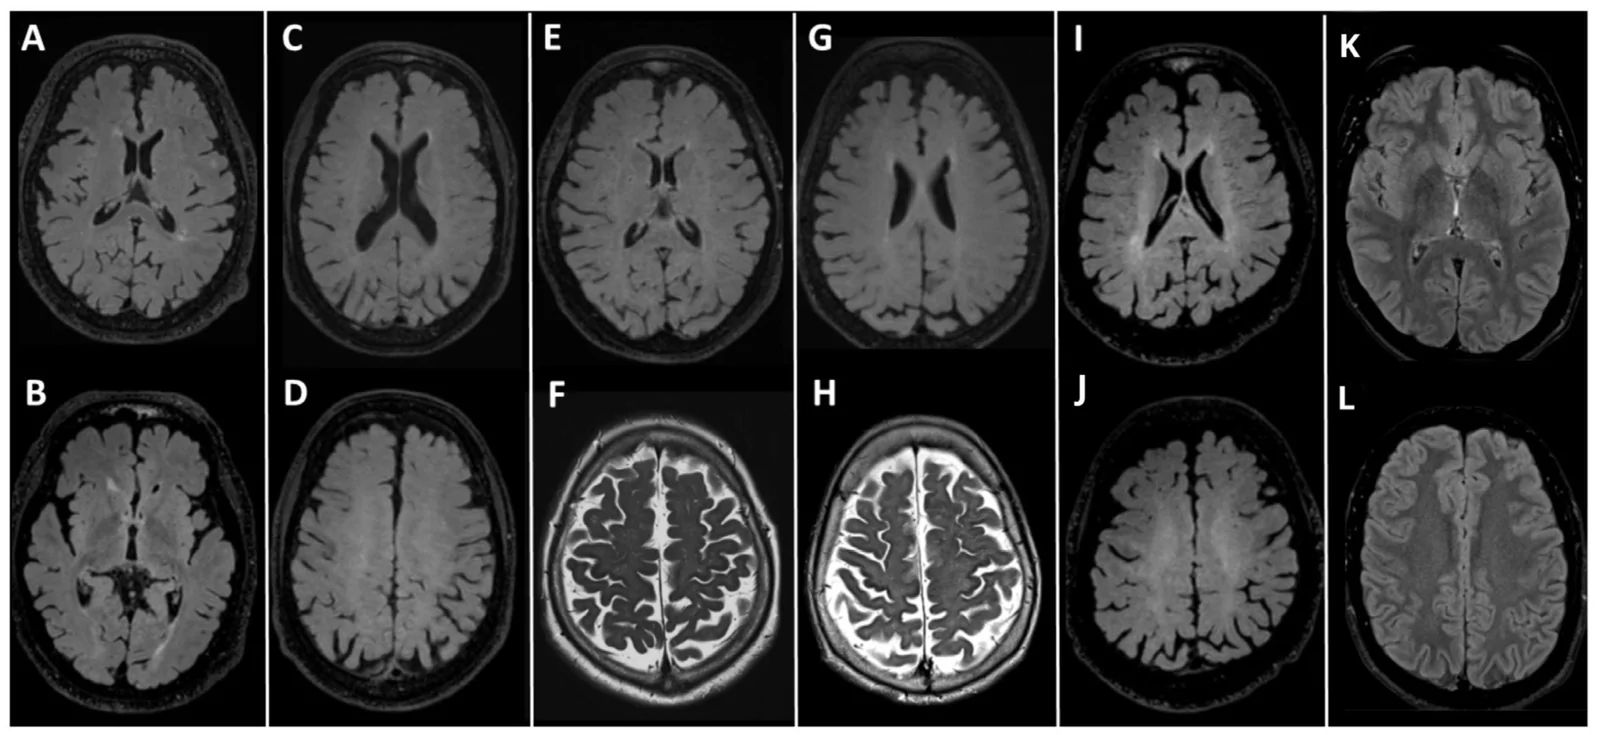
Axial FLAIR images demonstrate uniform white matter signal abnormalities with loss of normal gray-white matter contrast, consistent with hypomyelinating leukodystrophy in all subjects (A,B) subject 1; (C,D) subject 2; (E) subject 3; (G) subject 4; (I,J) subject 5; Axial FLAIR images (A,B,I,J) also show more pronounced symmetrical white matter hyperintensities with occipital predominance in two subjects; Axial T2-weighted images (F,H) demonstrate bilateral hypointensity in the Rolandic cortex in two subjects; note the normal gray-white matter contrast in FLAIR images (K,L) from a healthy subject, not carrying the variant, from the same family (son of subject 3).
